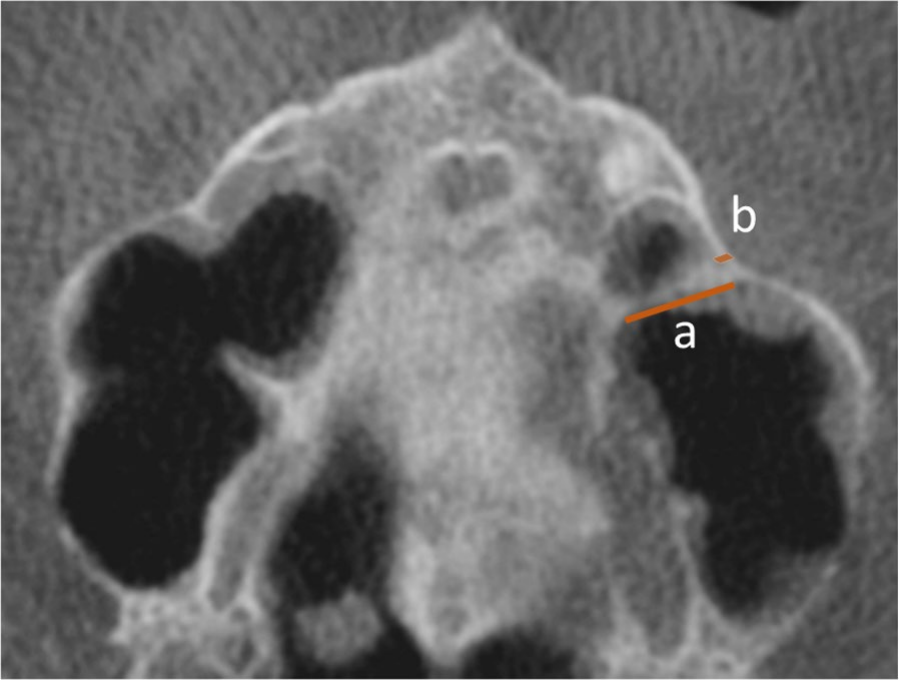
Cone-beam computed tomography (CBCT) image of the maxillary measuring the septum in the oro-vestibular extension (a) and the thickness of the buccal cortical bone (b): coronal slice
